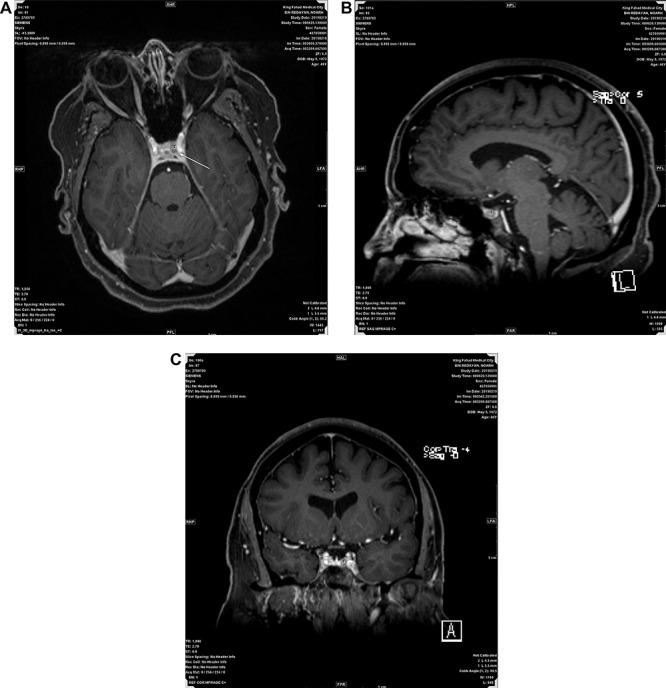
(A, B and C) Represent post contrast Axial, Sagital and Coronal MRI images respectively, showing a small non enhancing nodule in left side of adenohypophysis measuring 5.5 mm suggestive of microadenoma.
